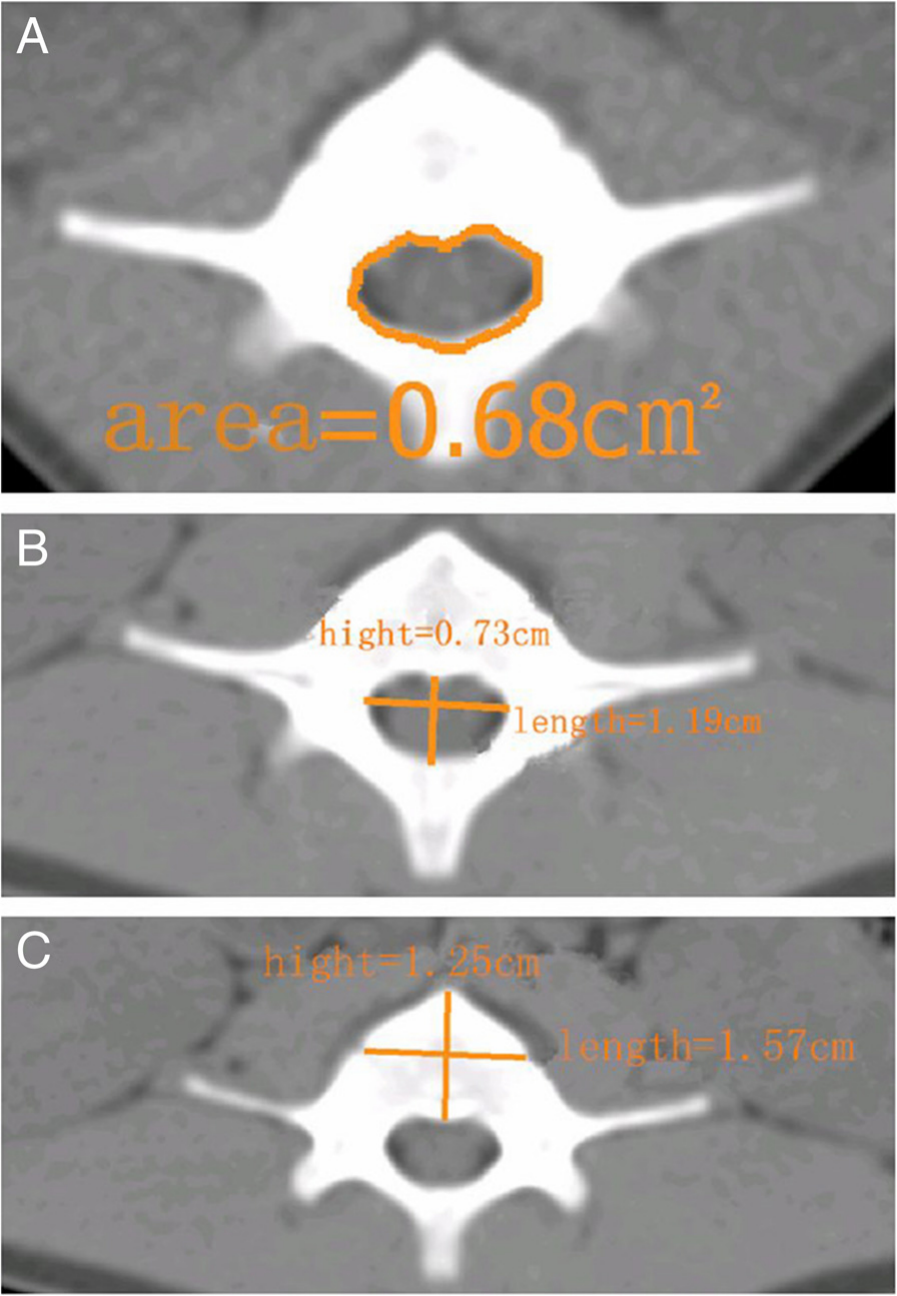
Images of CT are shown as examples of how the measurements of canal area (a), vertebral transverse/sagittal diameter (b), and canal transverse/sagittal diameter (c) were performed. Numbers in the images were typical readings for these measurements
